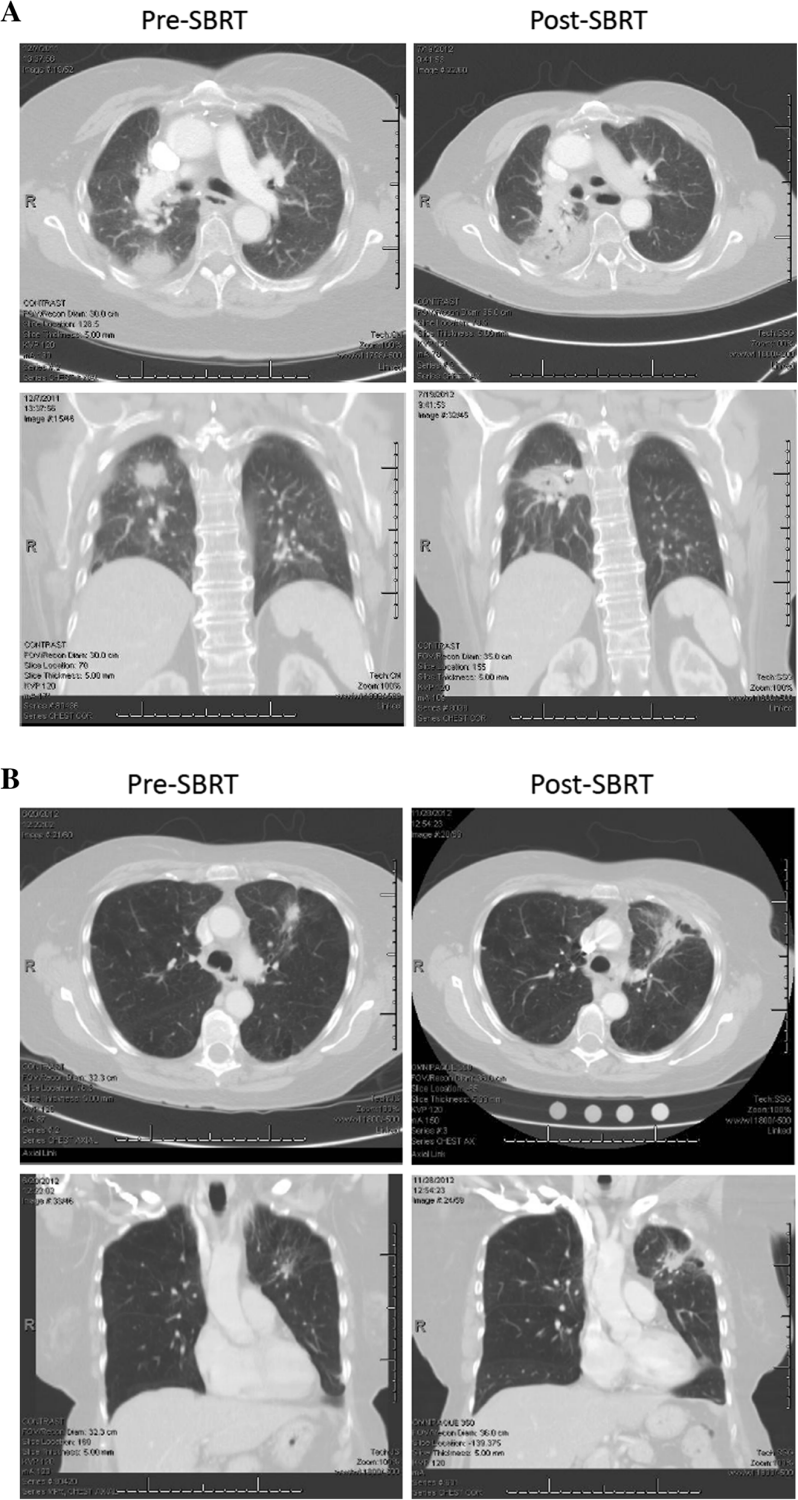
Radiation associated imaging changes. Panels A and B show typical radiation changes that are apparent on chest CT approximately 3–9 months following SBRT. Images on the left show pre-treatment tumor and images on the right show post-SBRT changes. Both axial and coronal planes are provided. The imaging changes depicted above are considered grade 1 radiation pneumonitis by the CTCAE v4.o criteria if they are not associated with clinical symptomatology.
